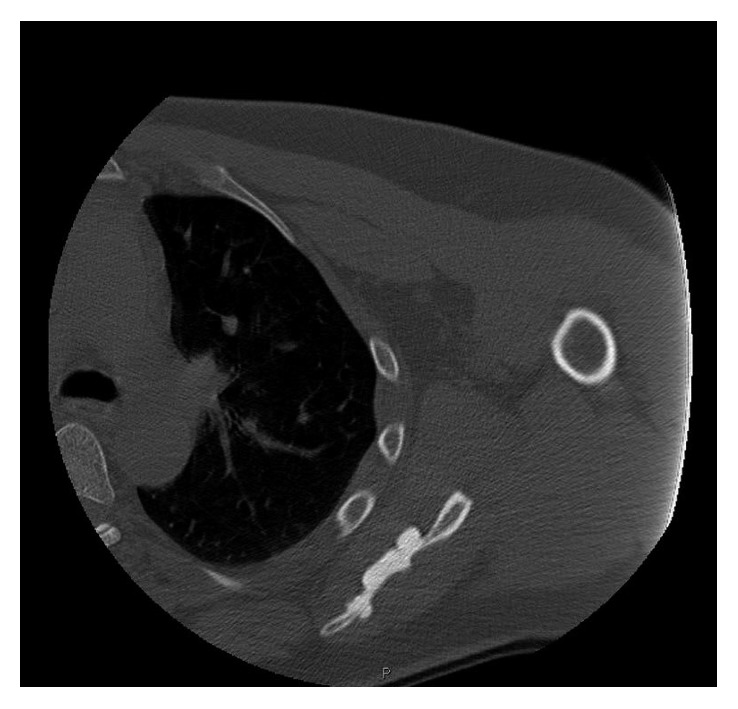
Computed tomography scan of the left shoulder. Axial cut showing hyperostotic masses dribbling from the scapular blade.
